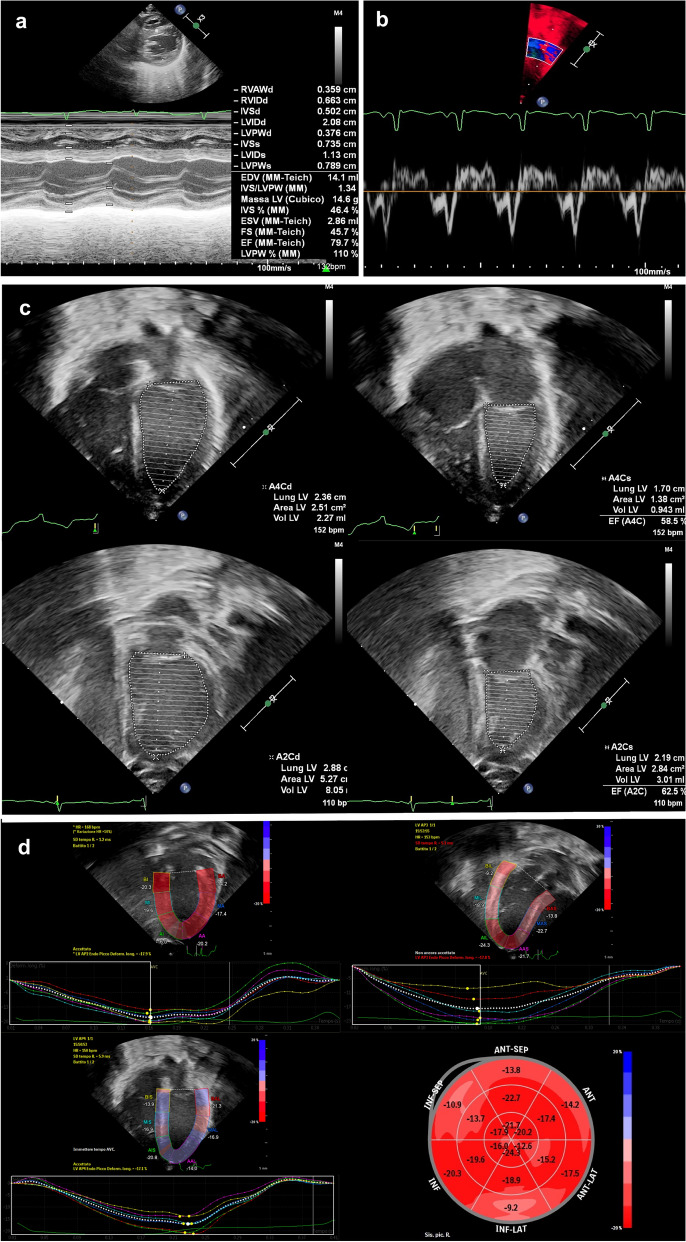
(a) M-mode image of a short axis view at the level just distal to the leaflet tips of the mitral valve showing shortening fraction (SF) calculation. (b) Tissue doppler imaging of an apical 4-chamber view with the sample volume at the lateral margin of the mitral valve, showing systolic peak velocity (S’) (c) Calculation of ejection fraction (EF) according to Simpson’s biplane method of disc. Apical 4-chamber views (top) showing the measurement of left ventricular end-diastolic (left) and end-systolic (right) volumes. Apical 2-chamber view (bottom) showing the measurement of left ventricular end-diastolic (left) and end-systolic volumes (right). (d) Longitudinal-Strain in 2-chamber view (top-left), 3-chamber view (top-right) and 4-chamber view (bottom-left). Bull’s-eye of global longitudinal strain (GLS). Bull’s eye plot derived from the longitudinal strain of the three apical views (bottom-right).
